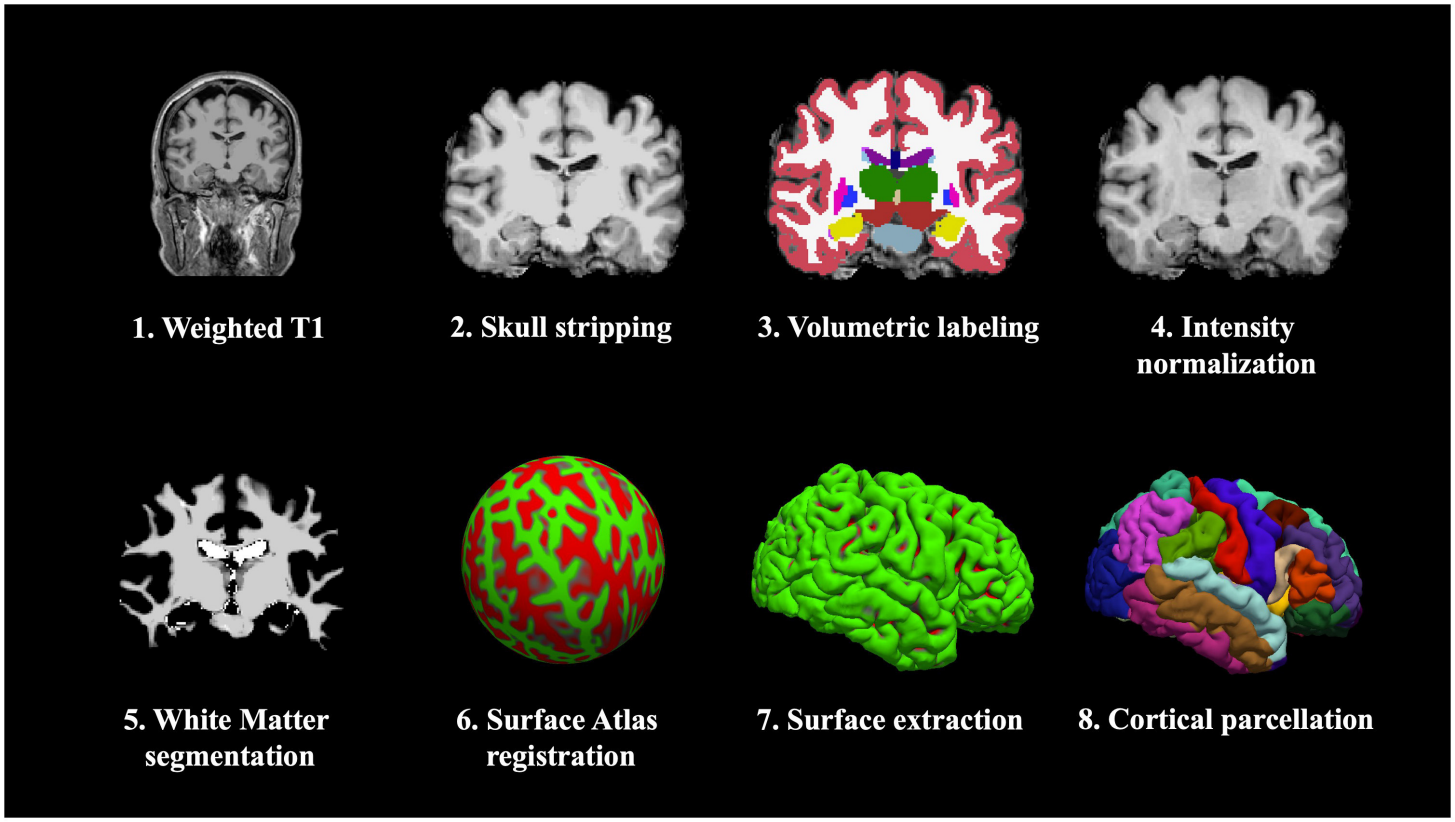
Brain MRI analysis process using FreeSurfer.
